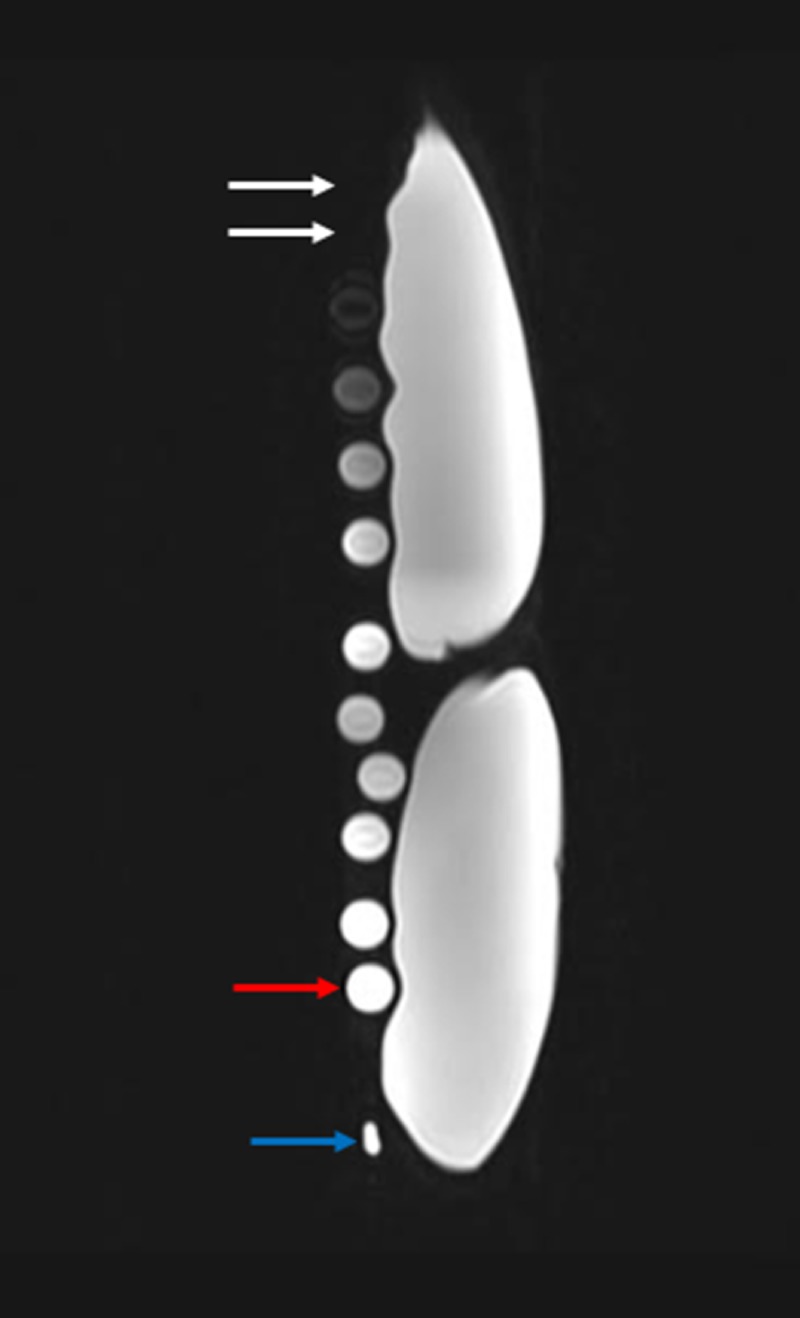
In vitro results of serial diluted gadolinium solution on T2 weighted magnetic resonance imaging The results of the in vitro experiment show the test tube with the most concentrated gadolinium solutions are not visualized, given the low signal intensity (white arrows). The test tube with the most dilute gadolinium solution (red arrow) has the highest signal intensity on T2 weighted magnetic resonance imaging. All test tube samples were imaged adjacent to two 500 milliliter bags of normal saline. The blue arrow represents the magnetic resonance imaging marker.
